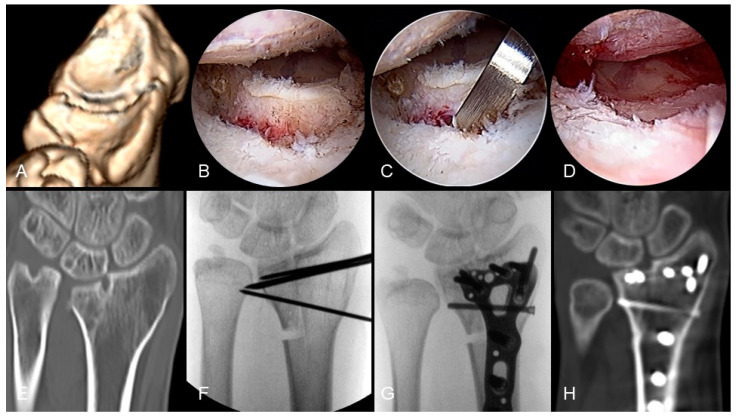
Arthroscope-assisted corrective osteotomy of intra-articular distal radius malunion: (A) preoperative articular step-off noted from 3D CT reconstruction with carpal bones subtraction; (B) articular step-off noted through arthroscope; (C) inside-out osteotomy; (D) reduction of intraarticular malunion; (E) preoperative CT coronal view; (F) temporary K-wires fixation of reduced articular surface; (G) definitive fixation and length correction with additional ulna shortening osteotomy; (H) follow-up CT coronal view 8 months later. Image courtesy of the corresponding author Chen-Yuan Yang, MD.
